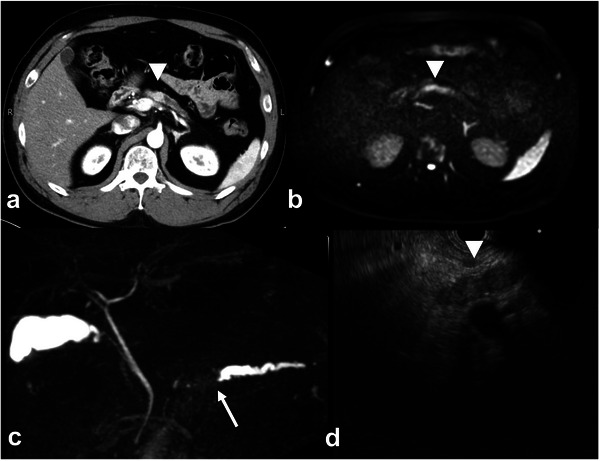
Pancreatic neuroendocrine tumor with intraductal growth into the main pancreatic duct at initial examination. Abdominal CT images showing a well‐enhanced mass in the pancreatic body during the arterial dominant phase (a). The mass protrudes toward the main pancreatic duct (MPD) of the pancreatic body, resulting in downstream dilation. On magnetic resonance imaging (MRI), the pancreatic neuroendocrine tumor (pNET) occupying the MPD revealed high signal intensity on diffusion‐weighted images (b). Magnetic resonance cholangiopancreatography demonstrates a dilated MPD (arrow) and a space‐occupying lesion within the pancreatic body with significant upstream dilatation of the duct in the body and tail of the pancreas, suggesting obstruction due to pNET (c). Endoscopic ultrasonography revealed a tumor filling the MPD within the pancreatic body. However, EUS could not determine whether the tumor originated within the MPD or from the pancreatic parenchyma (d).
